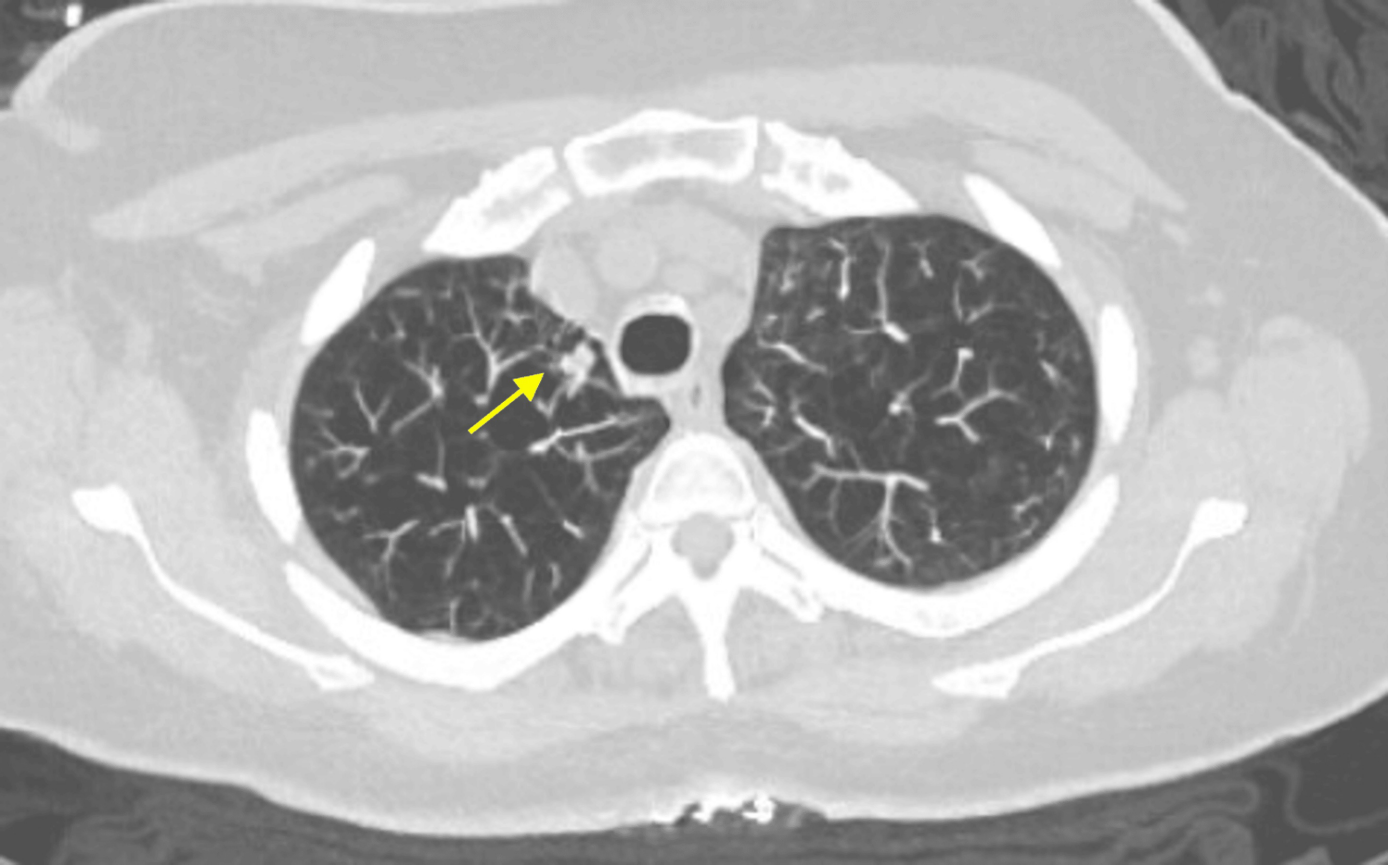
Chest CT scan in June 2024 showing a 7 x 7 mm nodule in the upper lobe of the right lung CT: computed tomography
